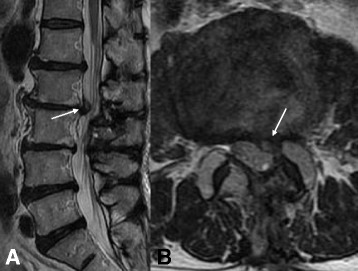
Magnetic resonance imaging shows recurrent upper lumbar disc herniation at the L2–L3 level. a Sagittal view. b Axial view. The *arrows* are pointing to recurrent upper lumbar disc herniation at the L2/3 level
